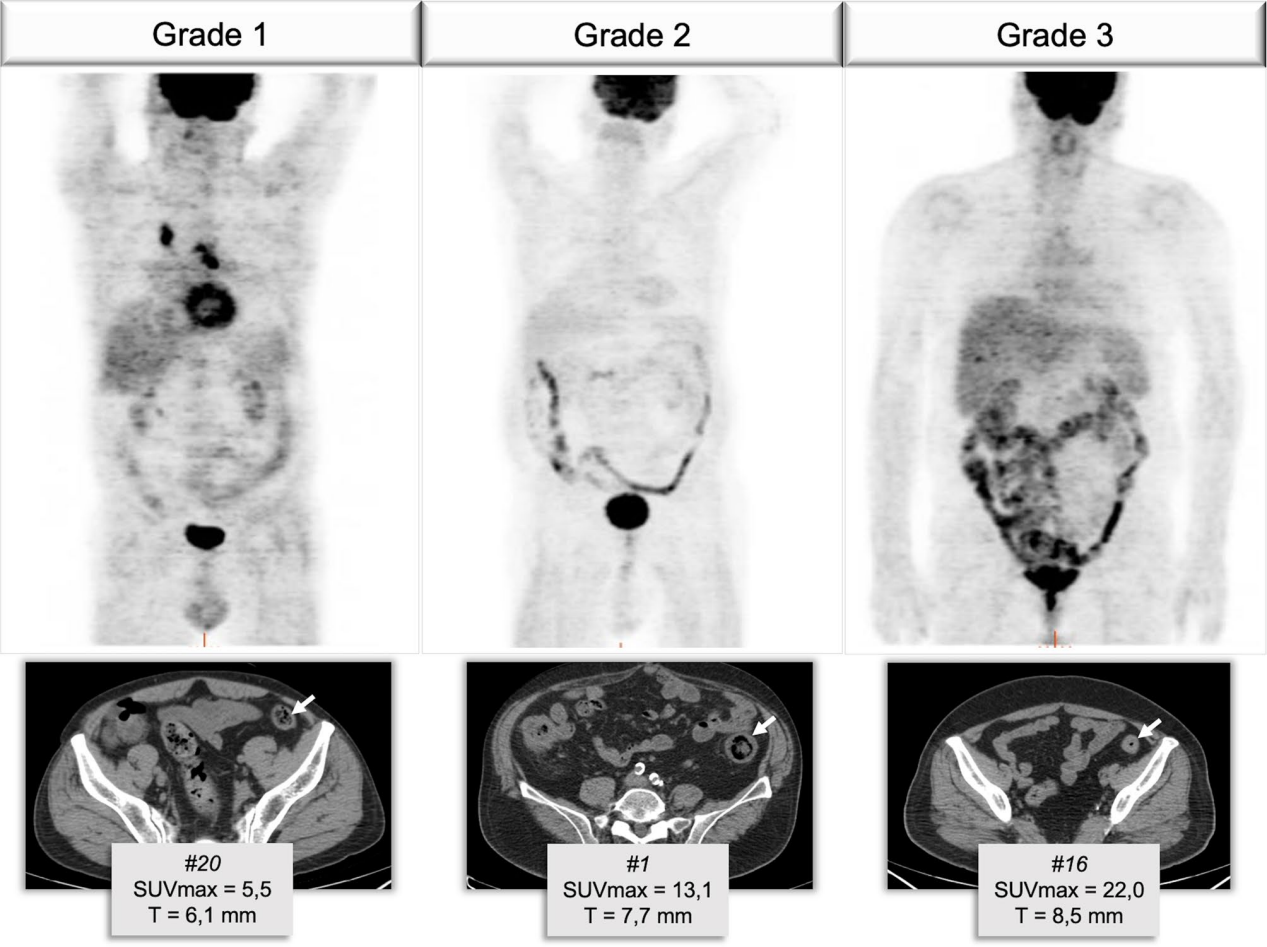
Exemplary cases of three patients who developed diarrhea with corresponding FDG colic uptake (expressed as maximum standardized uptake value - SUV_max_) and colic wall thickness diameter (T) measured on low-dose co-registered CT. The uptake as well as the colic wall thickness increase with the growing severity of the clinical irAEs
